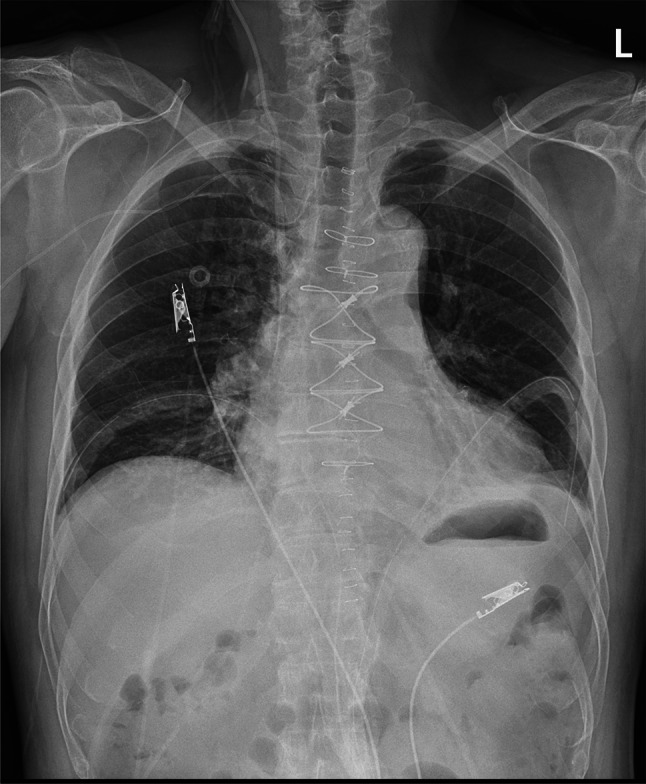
Two-pleural chest tube placement after OPCAB. Postoperative chest radiograph showing two chest tubes, one in each pleural space, with both tips directed toward the diaphragm.
